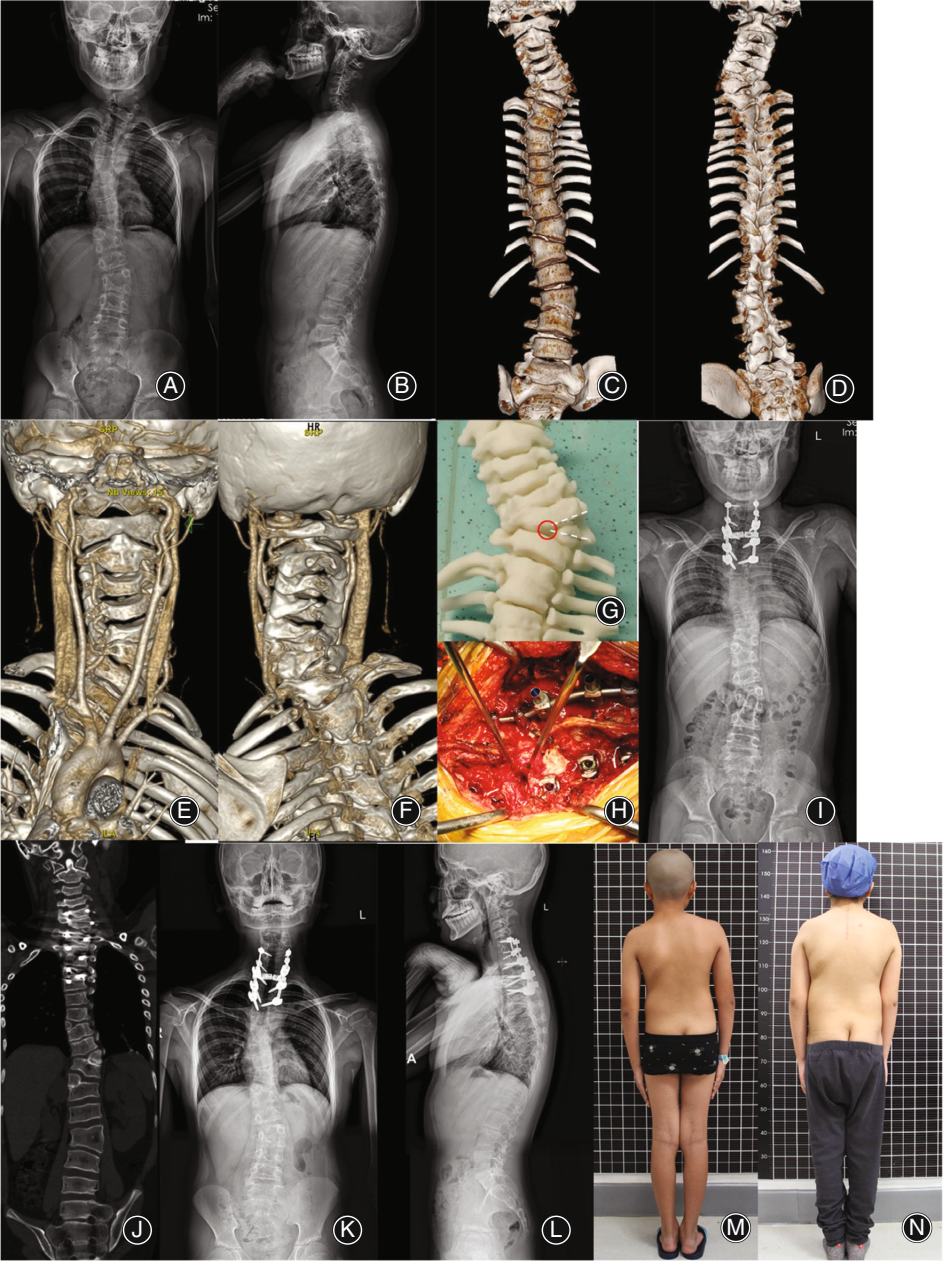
A 12‐year‐old boy with C7 hemivertebra and multiple malformations in the lower spine underwent a C7 hemivertebra osteotomy and internal fixation from C5‐T3 *via* one‐stage posterior surgery. (A, B) Preoperative X‐ray showed a main curve Cobb angle of 54.6° on the coronal plane, with a T1 tilt angle of 12.8°. (C, D, E, F) Preoperative CT and CTA showed a C7 hemivertebra with a large blood vessel around it and multiple malformations in the lower spine. (G, H) The C7 hemivertebra and superior intervertebral disc were resected by posterior approach surgery. (I, J) Postoperative X‐ray showed a main curve Cobb angle of 8.7° with, a correction rate of 87%. CT showed that the bone surface was closed after osteotomy. (K, L) X‐ray at the 2‐year follow‐up showed negligible loss of correction lost. (M, N) Comparison of the preoperative and postoperative appearance showed satisfying correction of the neck tilt and shoulder imbalance
